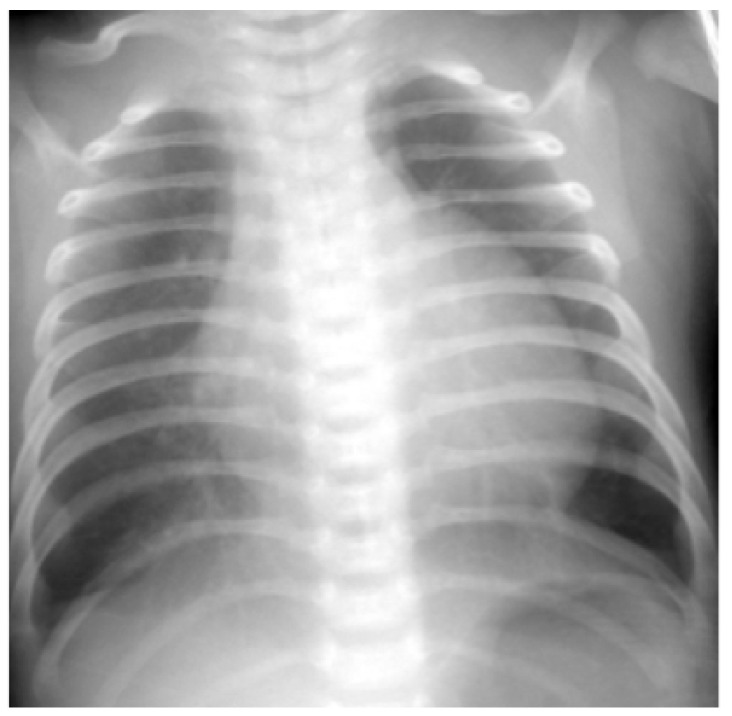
Chest radiography of a 52-day-old full-term male infant presenting with cyanosis and diagnosed with pulmonary hypertension showed bilateral hyperlucent lung and cardiomegaly with a cardiothoracic ratio of 0.64.
